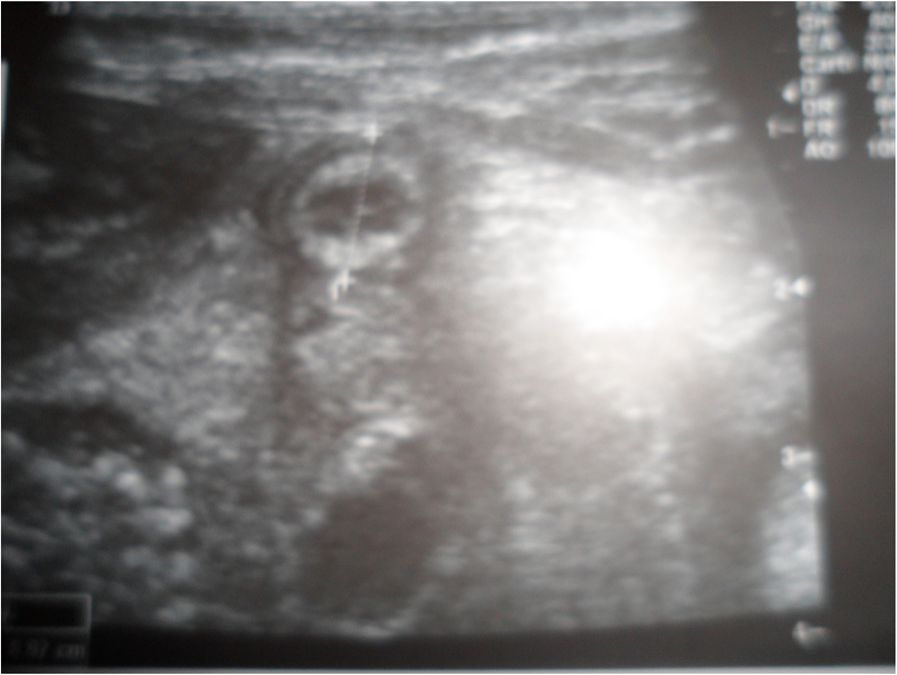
Abdominal ultra sonography of our patient showing appendicitis.
